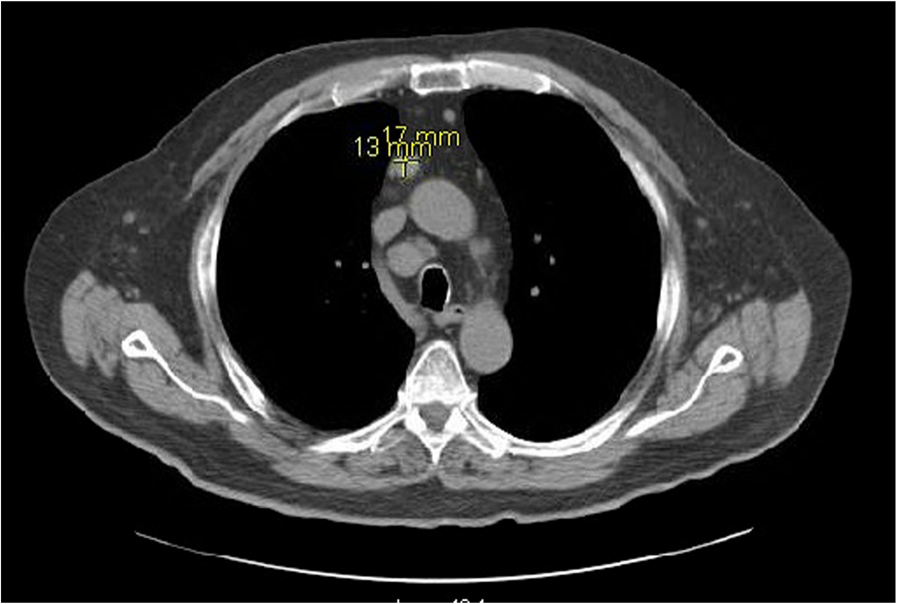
Computed tomography of the thorax showing the anterior superior mediastinal node measuring 1.7×1.3cm.
